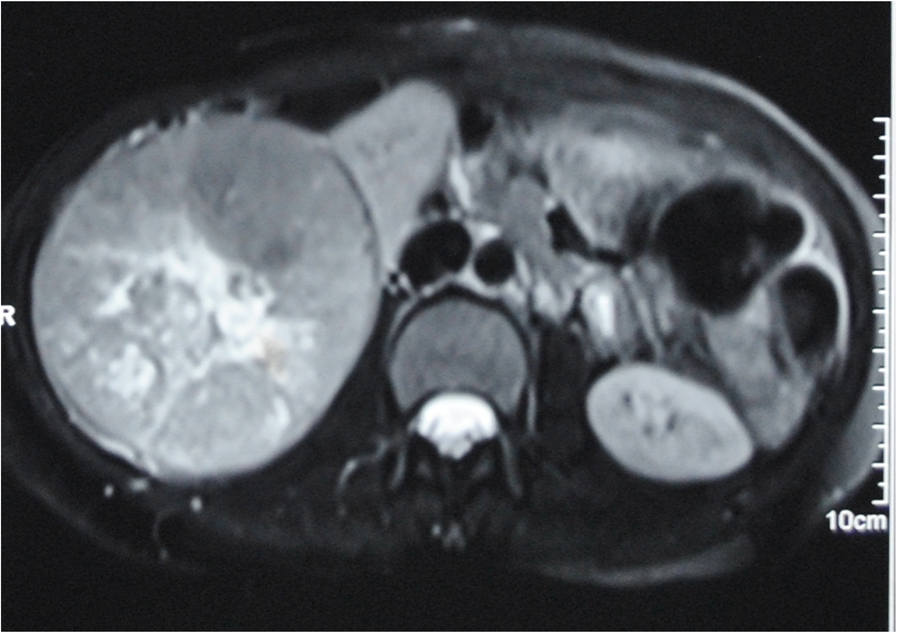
T2-weighted MR image showing a mass replacing whole of the kidney with no extracapsular invasion
